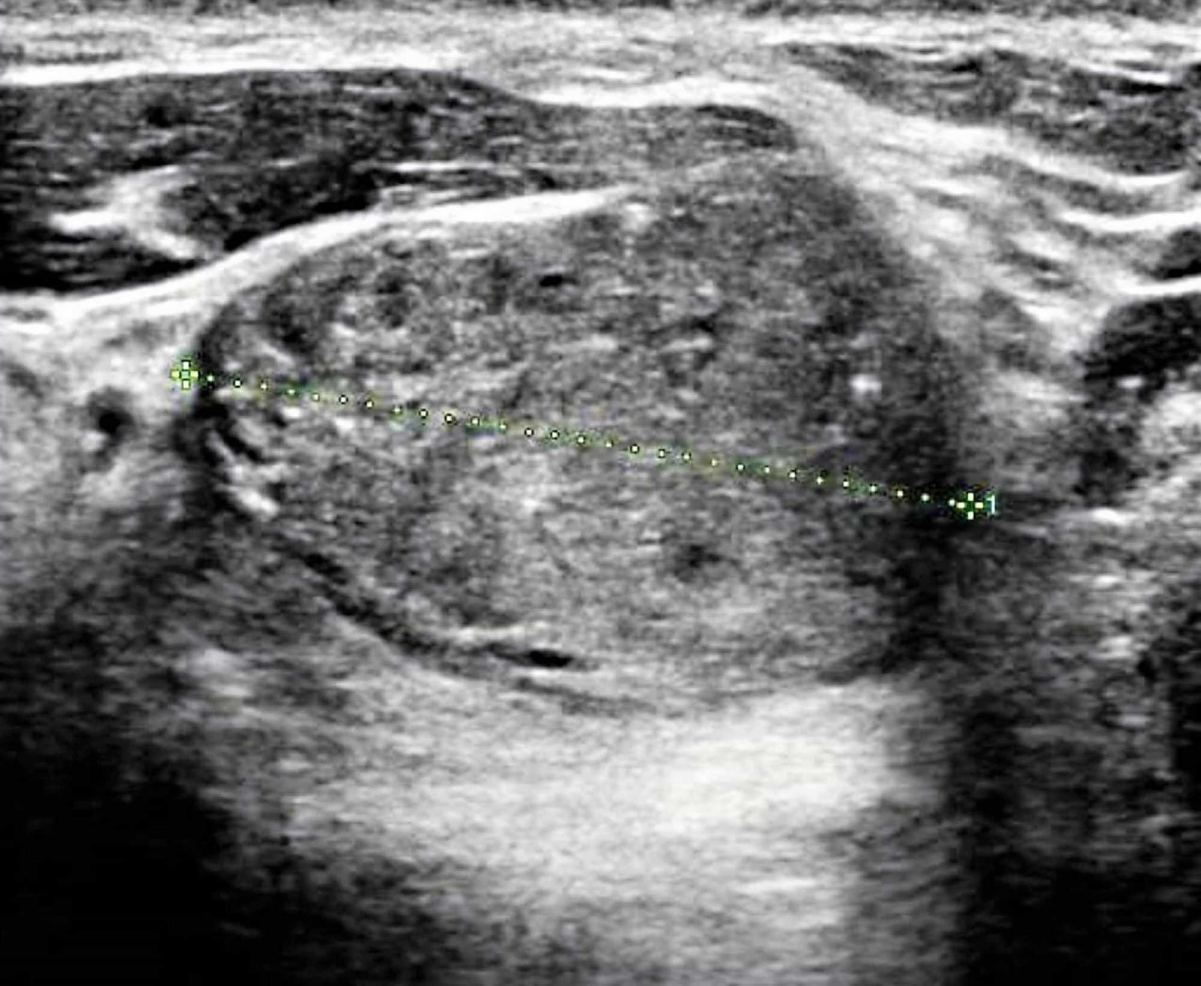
Ultrasound of the swelling shows a well-defined oval lesion around the medial end of the right clavicle (green dotted line)
